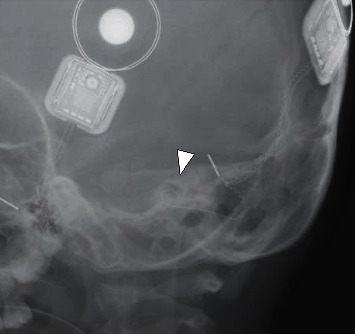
This is an intraoperative lateral skull X-ray taken after the electrode was advanced. The electrode resumed a similar position to Figure 2.
